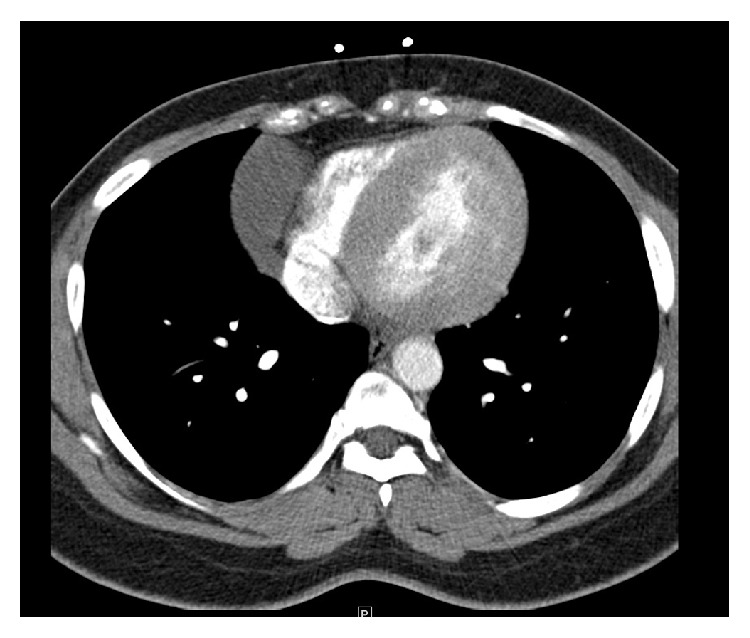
Coronary sinus atresia: absence of coronary sinus at the expected location on axial contrast CT chest image.
